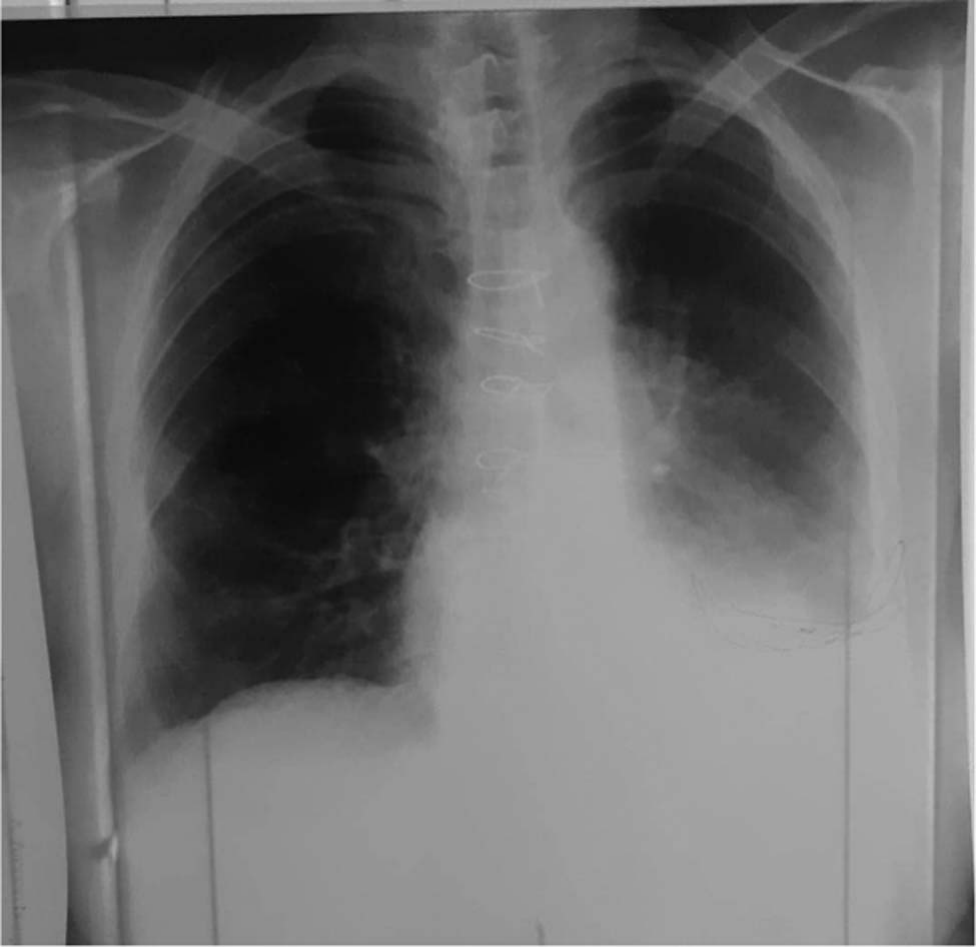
A follow-up chest radiography performed two weeks postsurgery showed normal findings.
